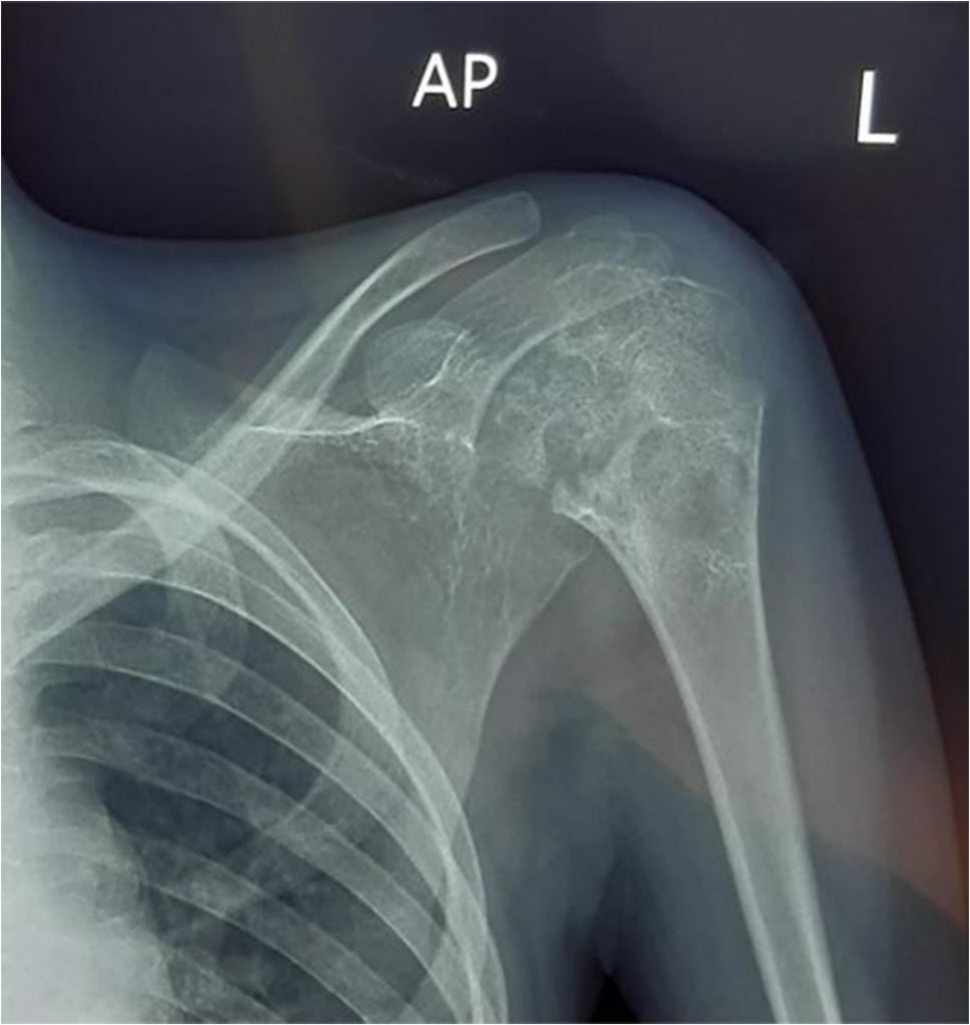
Anteroposterior shoulder radiograph in a 4-year-old girl presenting with left shoulder pain demonstrates the Phemister triad: juxta-articular osteoporosis, peripheral osseous erosions and narrowing of joint space, typical of tuberculous arthritis. Tuberculosis was confirmed on bone biopsy
